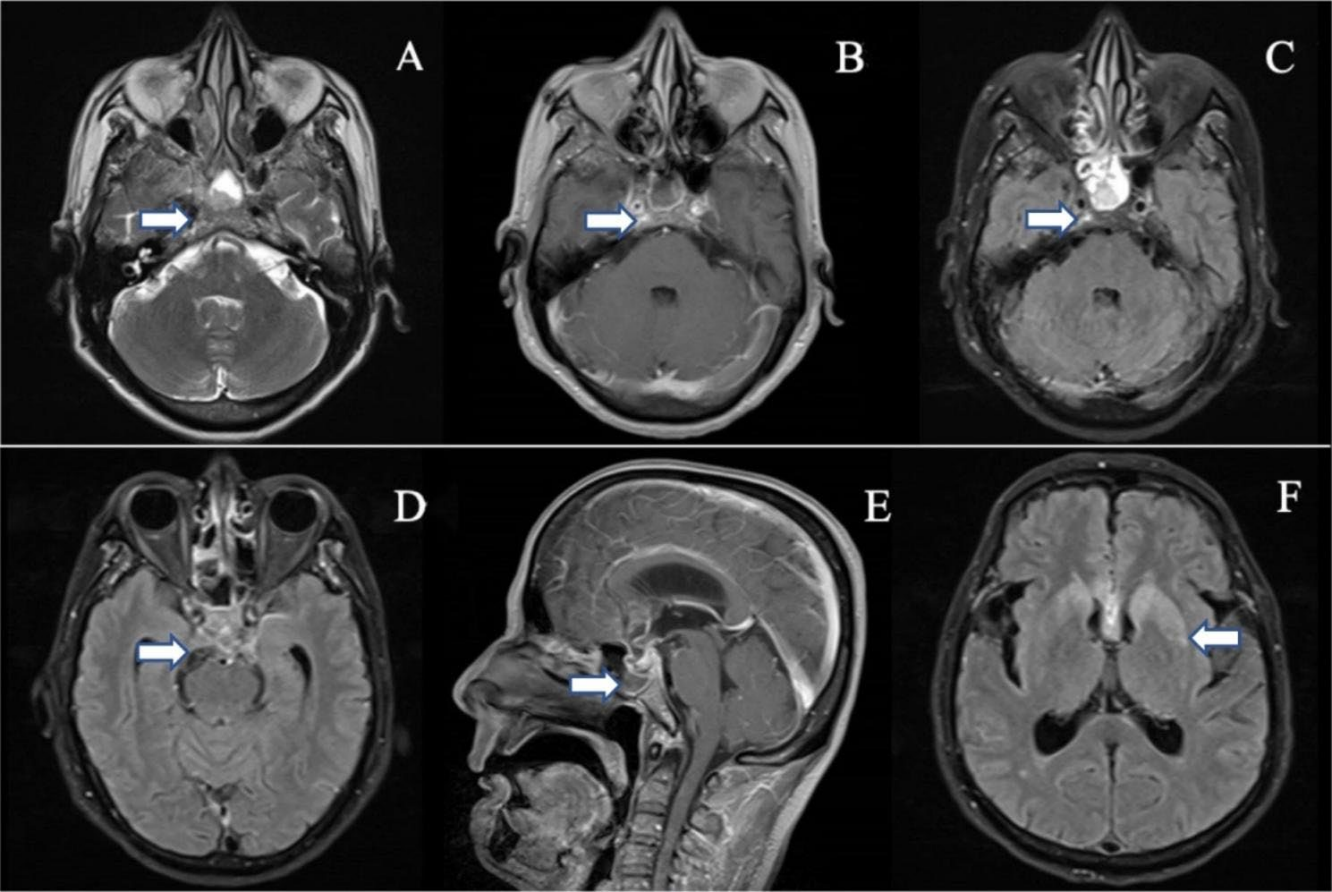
MRI of the brain: A: Slice of irregular and slightly long T2 signal in the sellar area. B, C: Slice of irregular enhancement changes in the sellar region. D: High signal in the flair sequence in the saddle region. E: Slice of irregular enhancement changes in the sellar and suprasellar regions. F: High signal in the flair sequence in the bilateral basal ganglia regions
